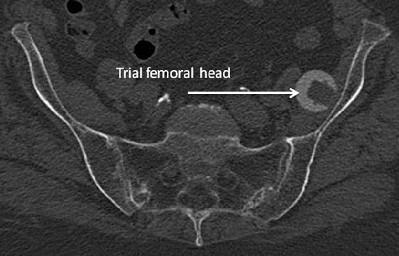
CT-Scan of prosthetic patient with lost femoral head, 5 days after surgery.
